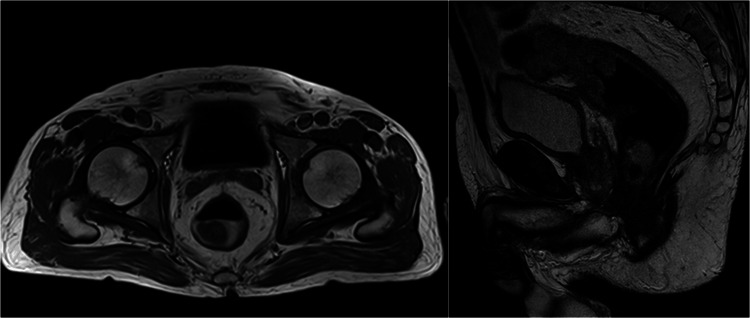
Magnetic resonance imaging revealed an 8 cm lesion at the rectosigmoid junction, with stenosis, perforation, and a vesical hypersignal.
